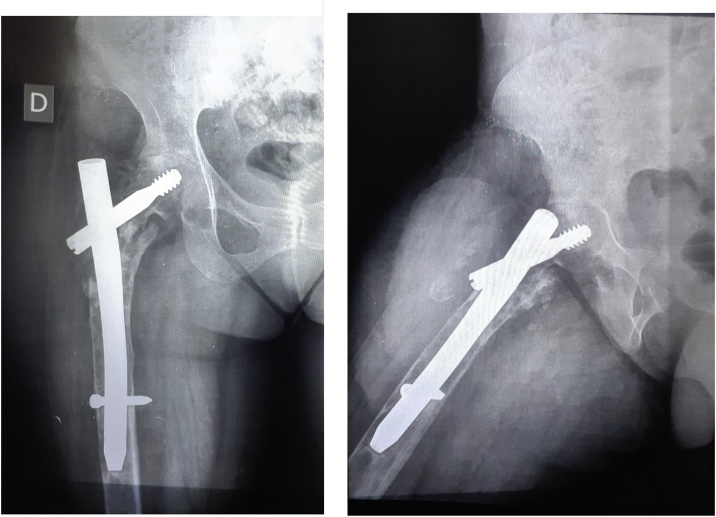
X-ray images showing the extensive lysis of the right trochanteric region and the femoral neck..
